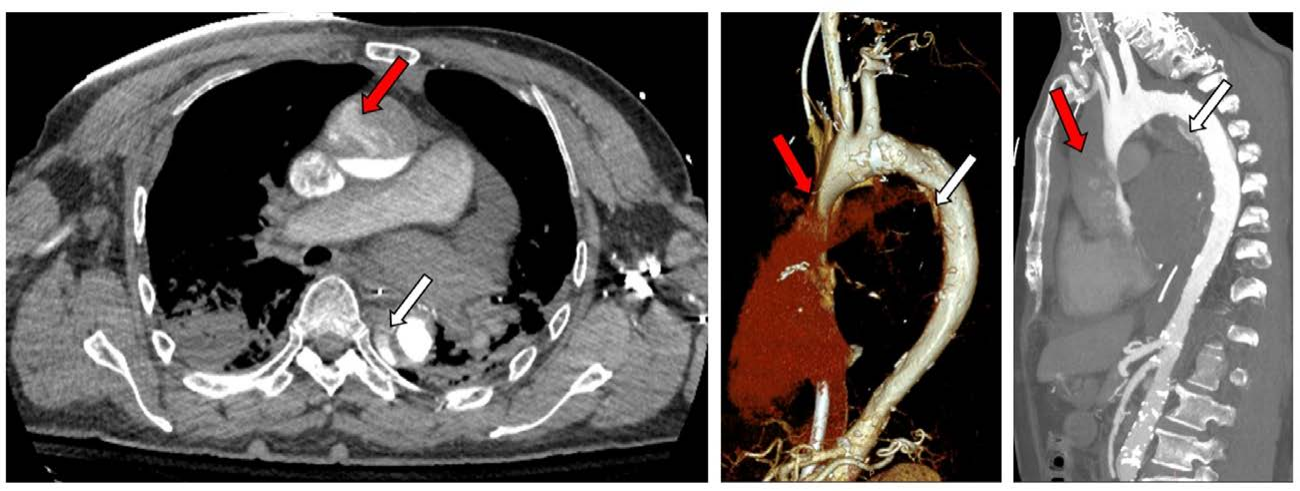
Aortic CTA showed aortic dissection without pericardial effusion. CTA = computed tomography angiography.
